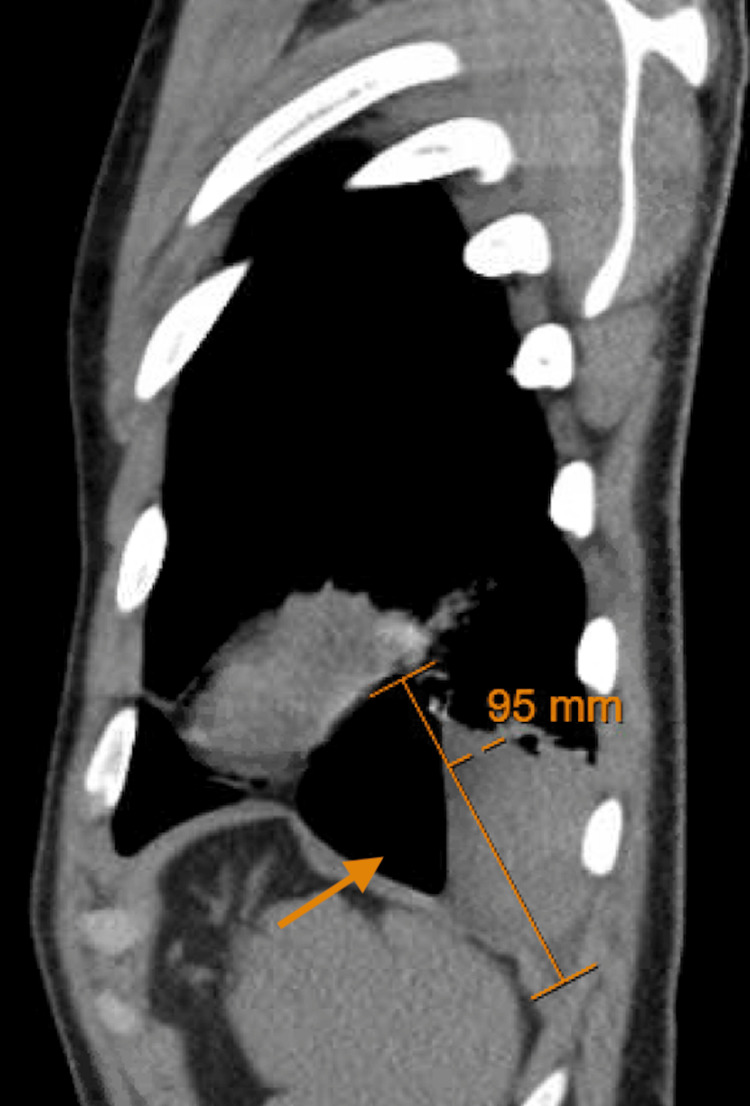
Sagittal CT scan of the chest showing a thin-walled cavitary lesion with an air-fluid level in the left lower lobe, as indicated by the arrow
